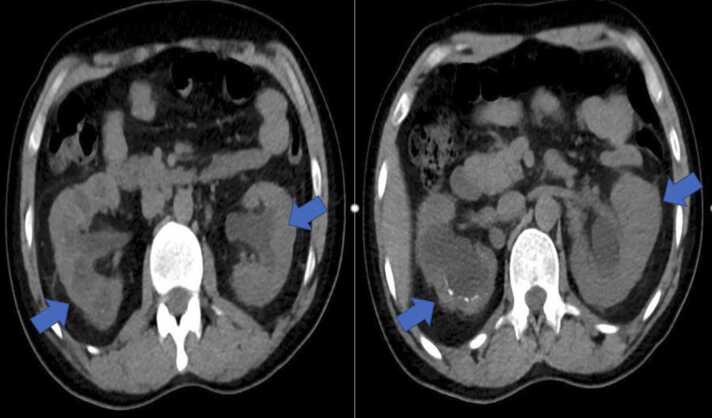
CT-KUB showing bilateral hydronephrosis with right kidney stones and perinephric fat stranding.
